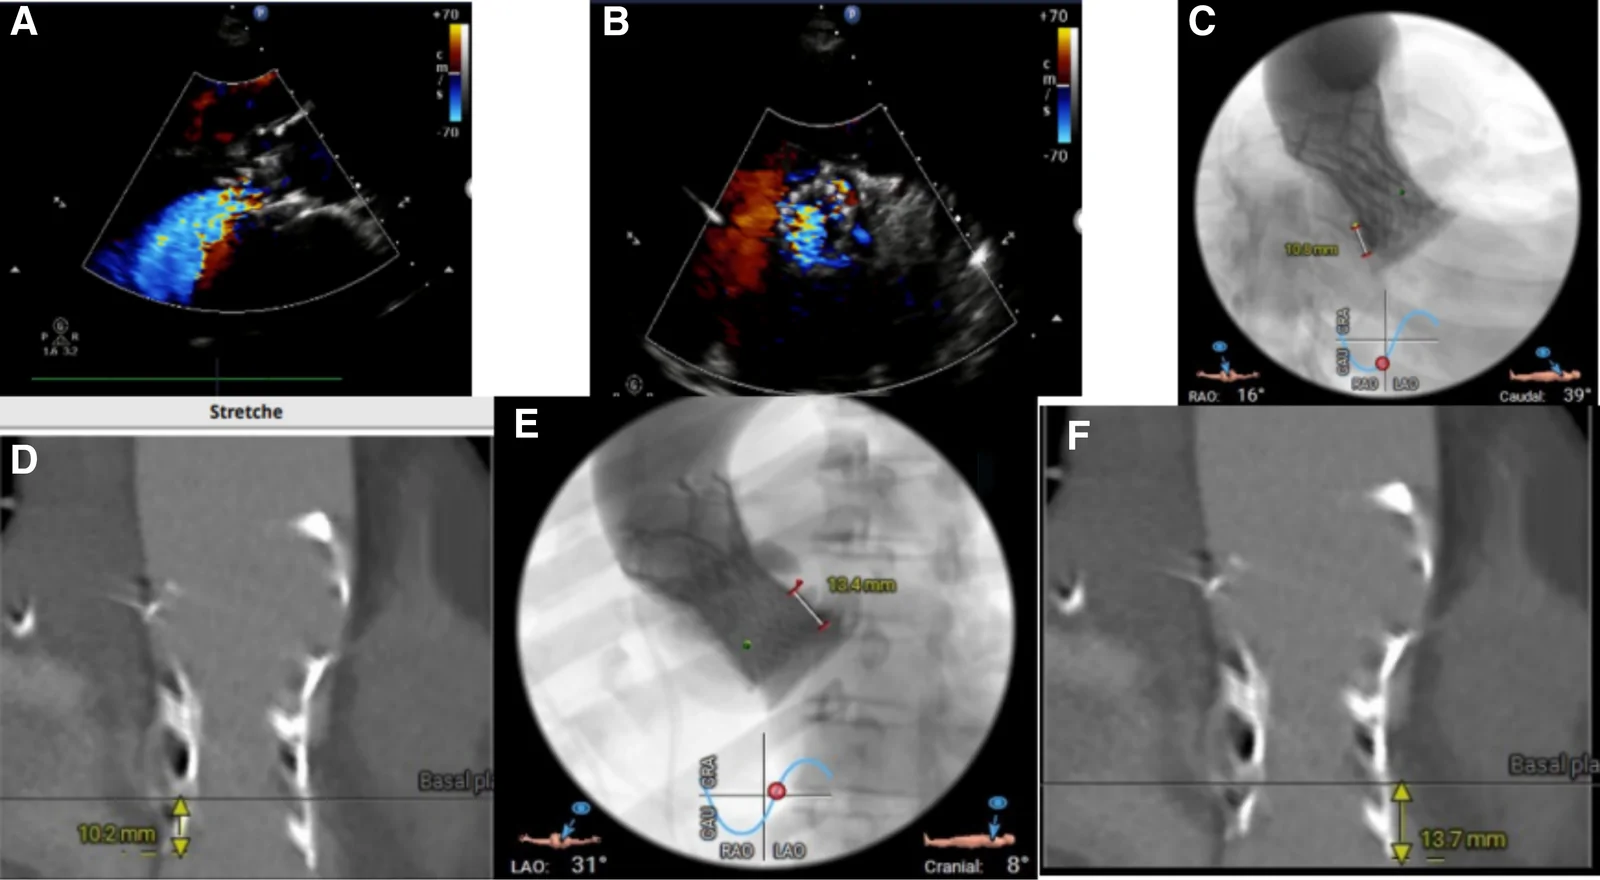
Bedside transthoracic echocardiography (TTE) evaluation and the relationship between the aortic root complications and the position of the bottom edge of the valve prosthesis and the virtual valve ring evaluated by 3-dimensional computed tomography (CT) before the 2nd transcatheter aortic valve replacement (TAVR) operation. The evaluation results of the long axis (A) and short axis (B) of the bedside TTE showed moderate and severe central regurgitation of the aortic valve and severe perivalvular leakage (PVL). At the tangent position of the valve, CT was used to measure the distance from the bottom of the valve prosthesis to the plane of the valve ring; the results showed 10 to 11 mm (C and D) on the side of the non-coronary sinus and 13 to 14 mm (E and F) on the opposite side.
